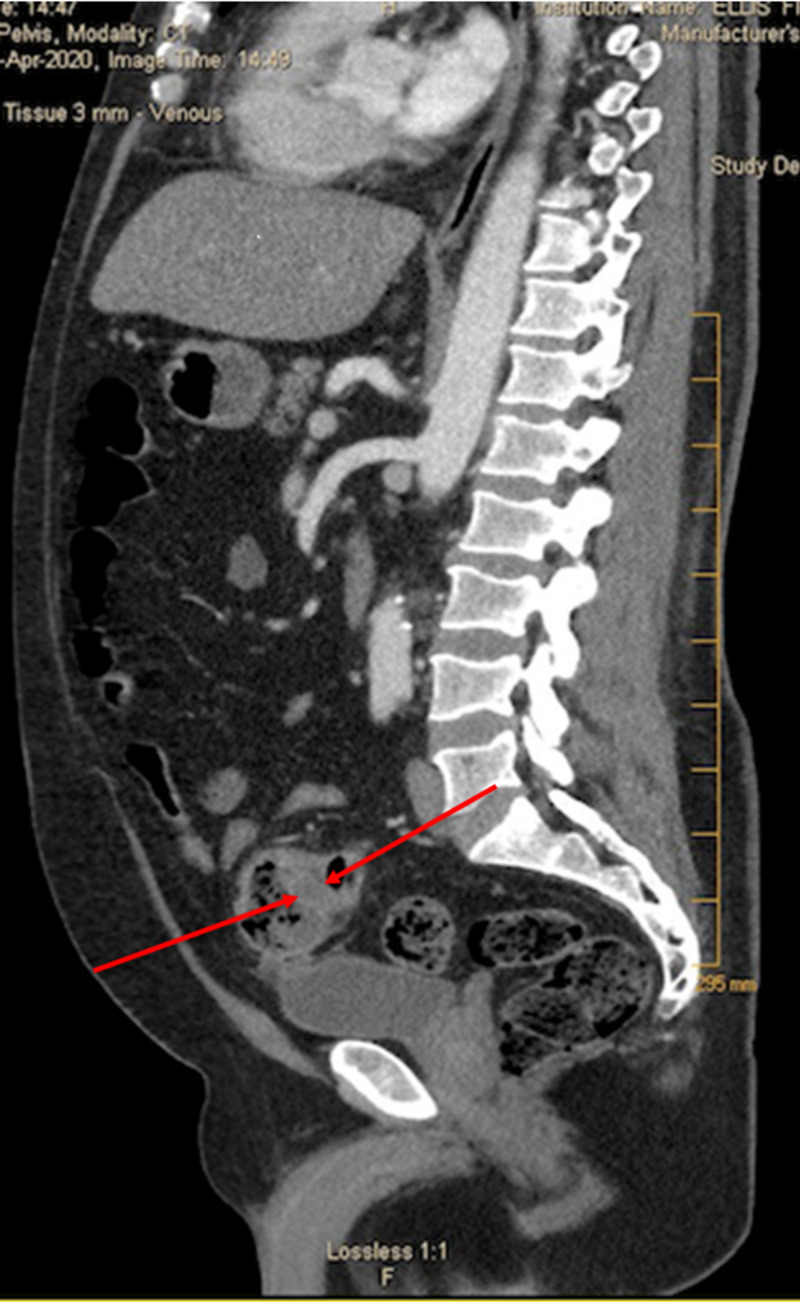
CT scan the abdomen, and pelvis revealed sigmoid diverticulitis and an adjacent small contained pericolonic abscess with dimensions of 3.5 x 4.3 x 4.1 cm.
